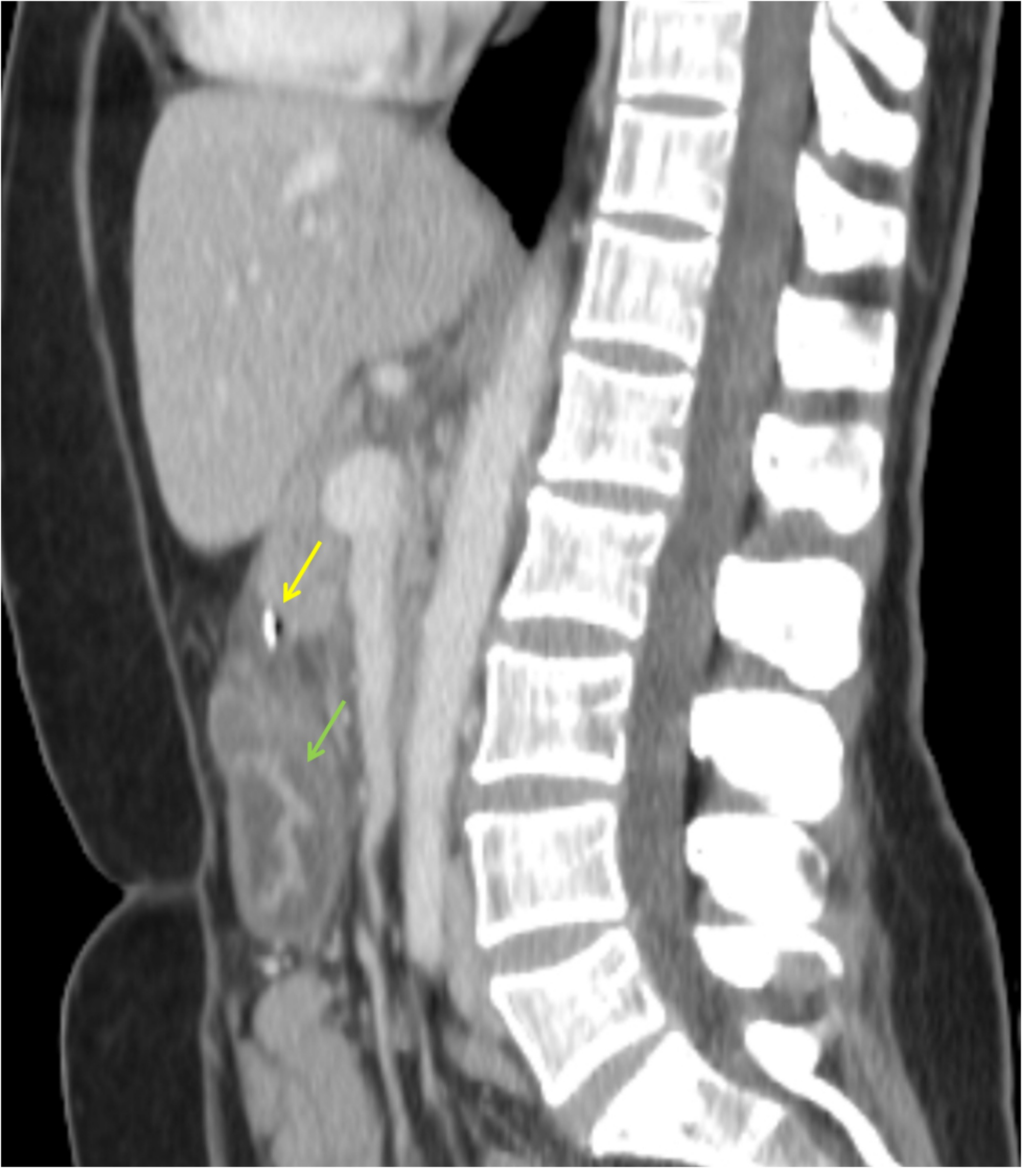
Abdominal CT: sagittal reconstruction The same patient in the sagittal view, with the yellow arrow pointing the fish bone and the green arrow showing thickened gastric walls due to local inflammation.
